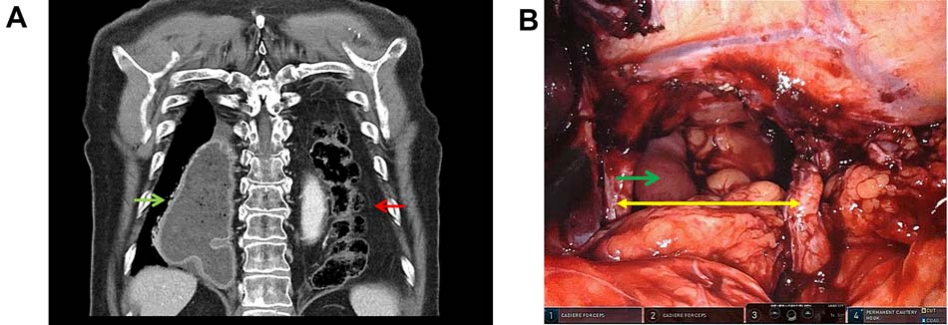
(A) Coronal image from the computed tomography demonstrating herniated colon into the left chest (red arrow) with compression on the dilated gastric conduit (green arrow). (B) Surgeon’s robotic console display with the endoscopic surgical view of the diaphragmatic hernia (yellow arrows) and the gastric conduit (green arrow), after reduction of the herniated colon.
